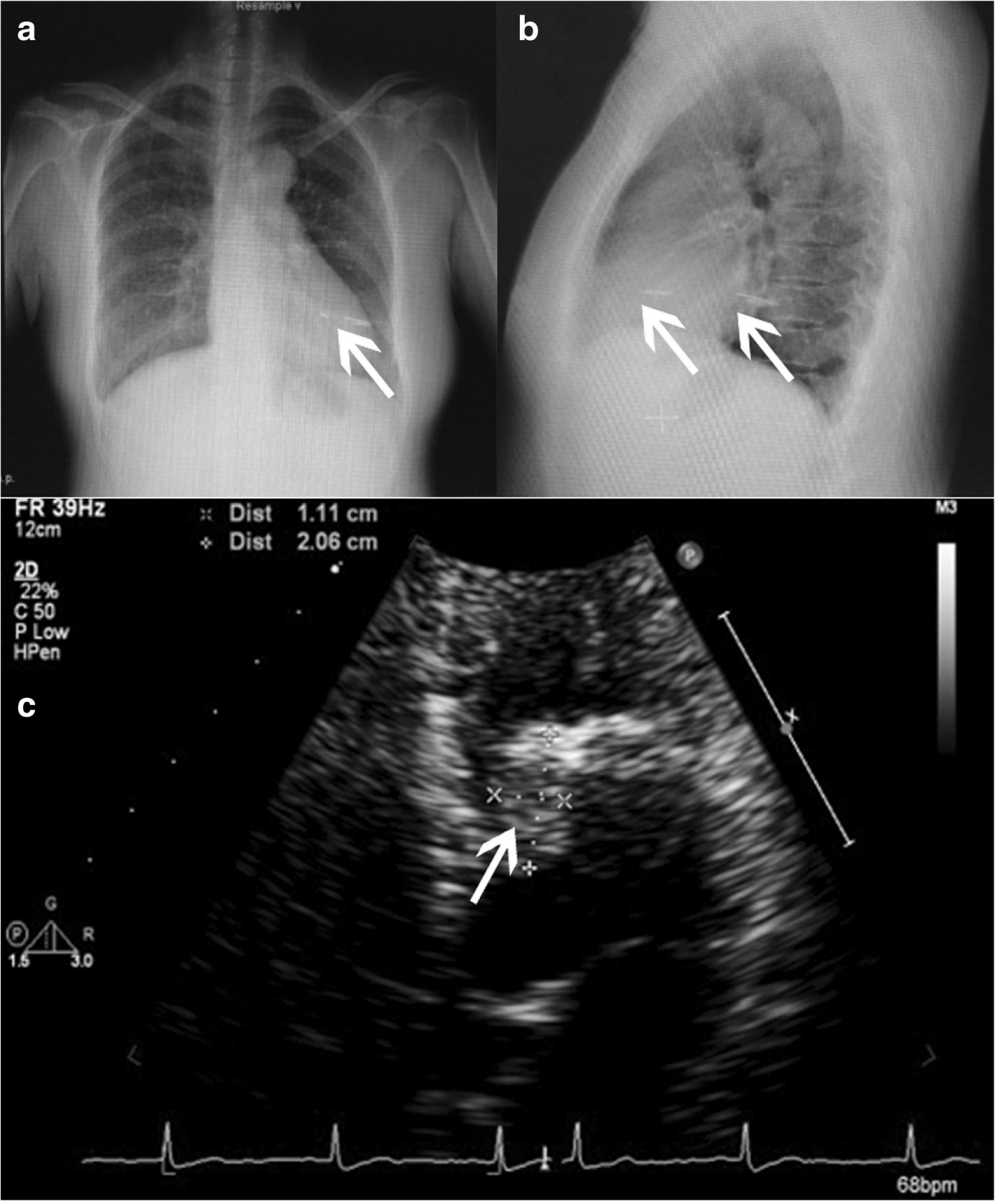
Chest x-ray and echocardiogram of the patient. a and b Chest x-rays show a needle in the heart and lung, respectively (*white arrows*). c Echocardiogram shows a huge echogenic mass (21 × 11 mm; *white arrow*) with some calcifications
